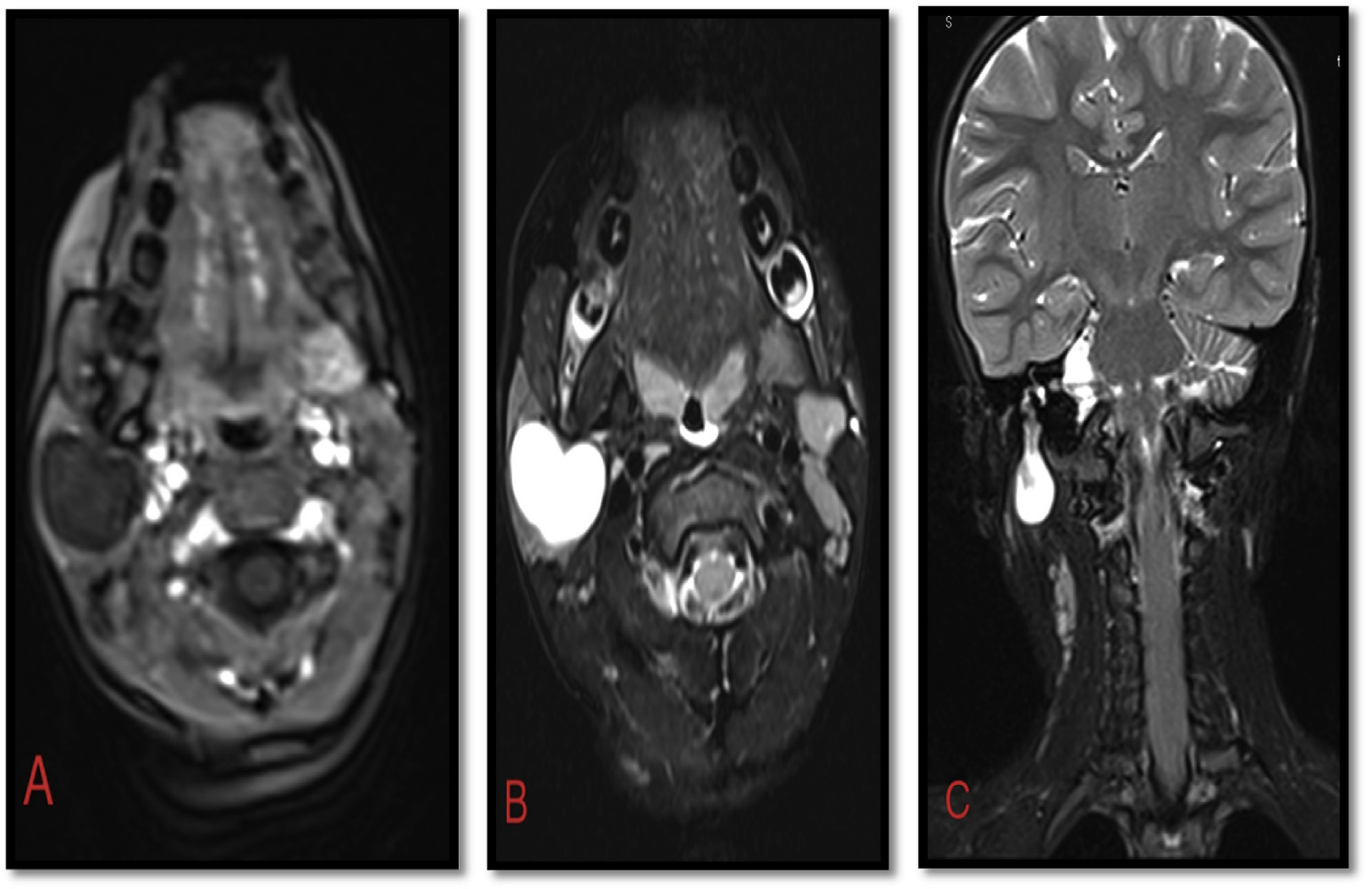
MRI of the parotid gland in sequence T1 (A) axial, T2 (B) axial, and T2 (C) coronal, showing the presence of a polylobed mass with a liquid signal, with an extension towards the skull base most likely related to the facial nerve.
